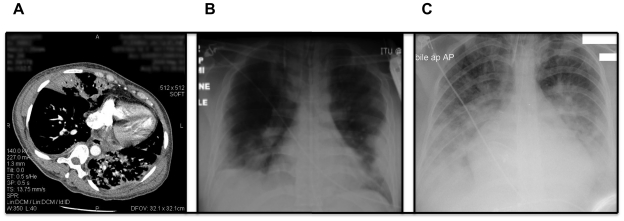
Radiographic images at presentation for Patient 1–3. Cut (mediastinal window) from mid thoracic region of the CT pulmonary angiogram of Patient 1 showing bilateral widespread nodular consolidation (A). The CTPA was reported as showing extensive inflammatory change in both lungs with solid consolidation of both lower lobes and right middle lobe; with widespread patchy infiltration in both upper lobes. CXR for Patient 2 (B) on day of intubation showed bilateral mid and lower zone consolidations, and possible left pleural fluid collection; and for Patient 3 (C) – extensive right lower lobe consolidation and patchy consolidation in left lower lobe; enlarged cardiothoracic ratio and evidence of pulmonary edema.
